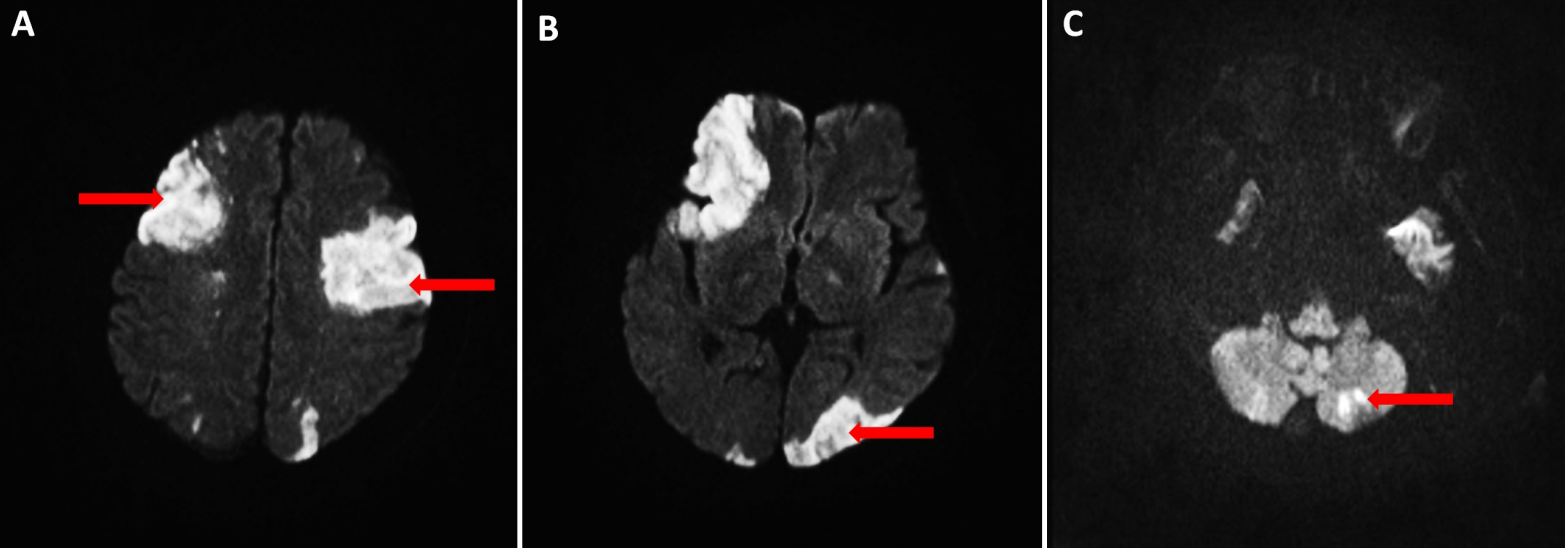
Magnetic resonance imaging of the brain (A-C) on day 18 after admission Axial diffusion-weighted images showing several areas of restricted diffusion (red arrows) within the middle cerebral artery territories bilaterally (A), in the left posterior cerebral artery territory (B), and in the left posterior inferior cerebellar artery territory (C), in keeping with acute infarct and suggestive of a cardioembolic etiology
